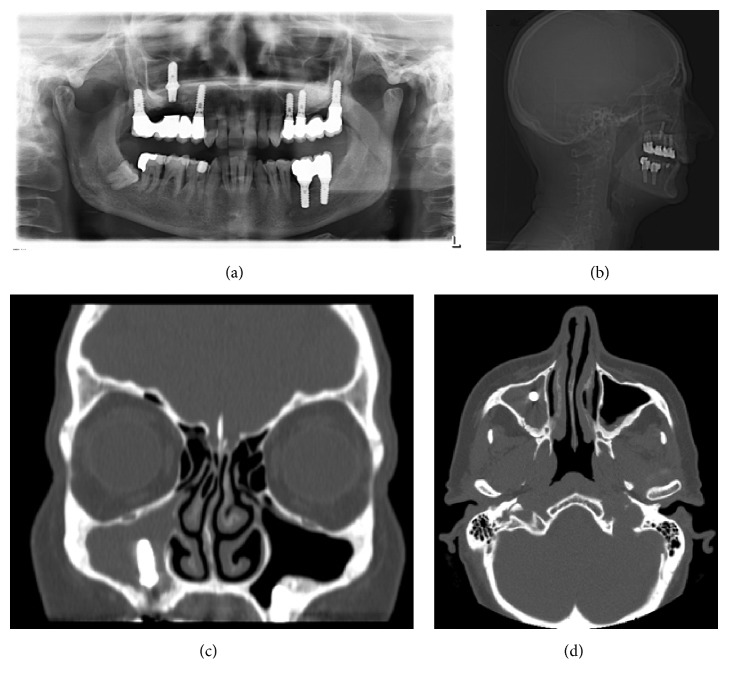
(a) Preoperative panoramic, (b) lateral cephalogram, and (c, d) CT scan radiographies showed the migration of dental implant sited in 1.6 positions into right maxillary sinus and clear signs of sinusitis.
